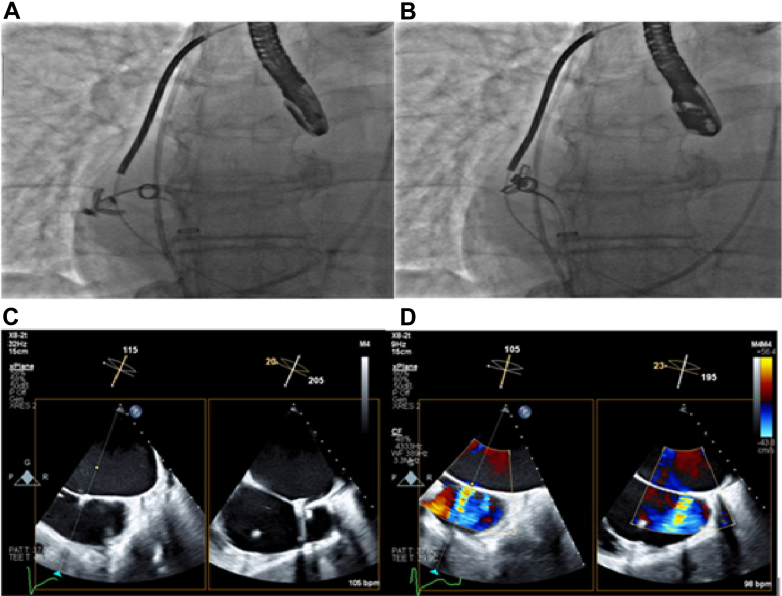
Fluoroscopic and Echocardiographic Images Depicting Events During Retrieval of Embolized MitraClip From Right Lower Pulmonary Vein (A) Fluoroscopic image showing a 10 mm gooseneck snare through multipurpose catheter directed at the embolized MitraClip. (B) Fluoroscopic image showing the gooseneck snare securely holding the neck of the MitraClip and pulled inside the steerable catheter. (C) Two-dimensional echocardiogram showing the MitraClip at the tip of the catheter and the snare assembly being pulled across the interatrial septostomy. (D) Two-dimensional TEE with color Doppler showing iatrogenic atrial septal defect after the clip and catheters have been pulled out of the interatrial septostomy. TEE = transesophageal echocardiography.
